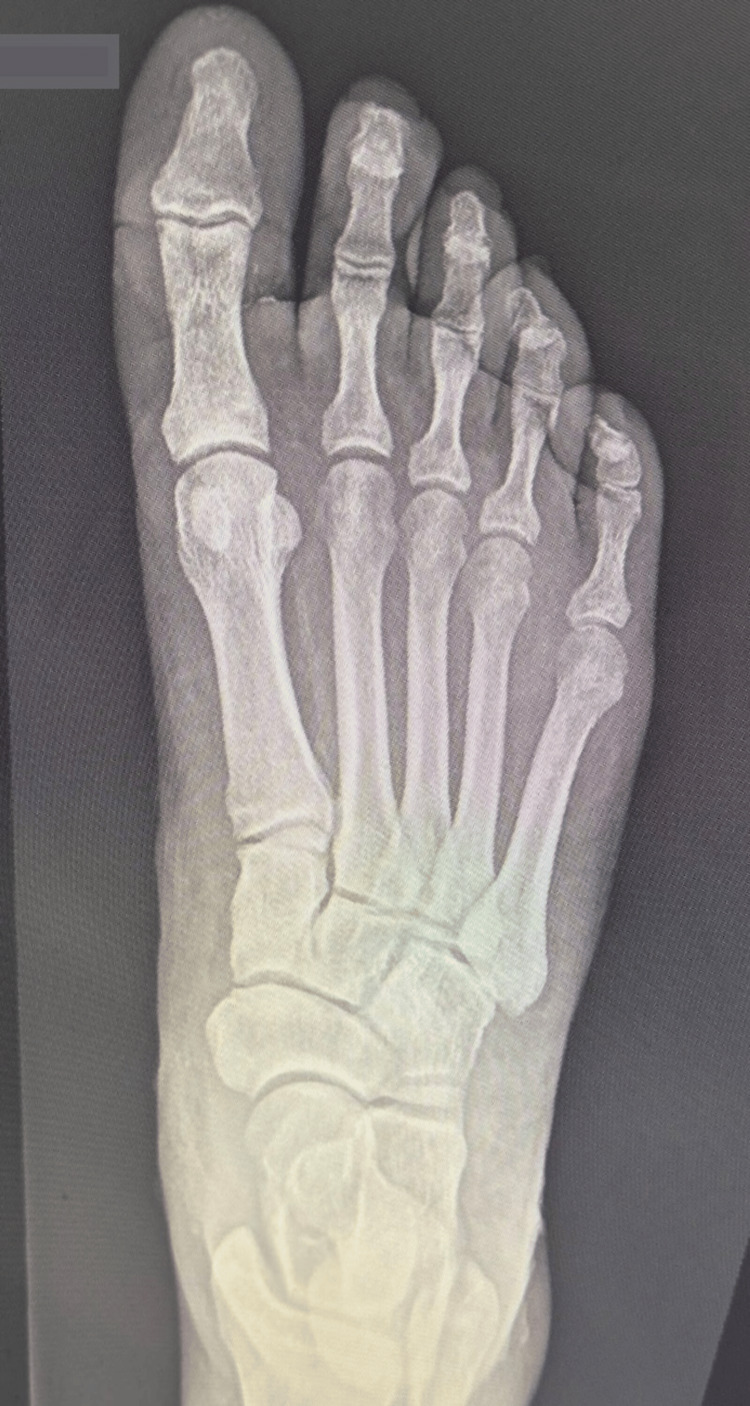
Dorsoplantar right foot X-ray with no signs of air pockets
